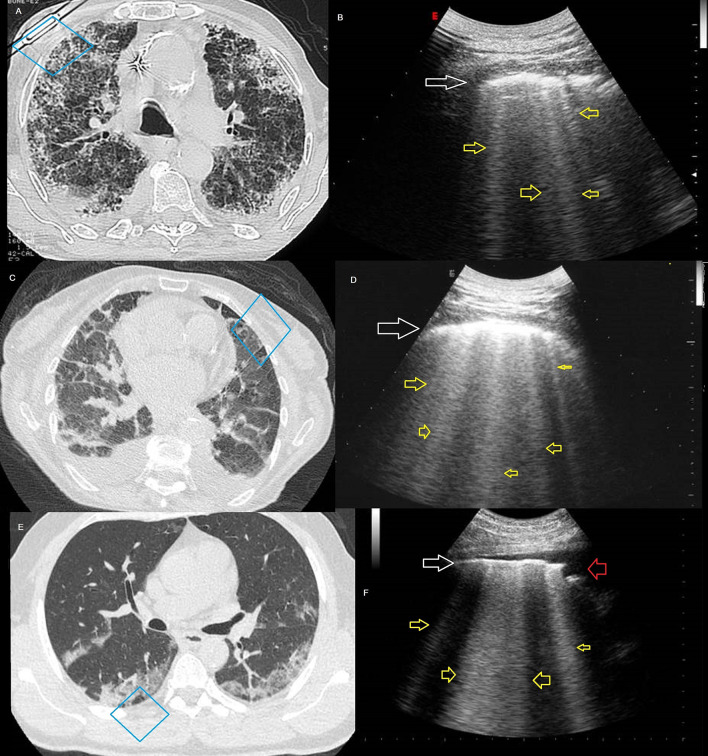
(A) Axial high resolution (HR) CT imaging showing a pattern of non-specific interstitial pneumonia (NSIP). (B) Ultrasound scan (corresponding to the blue box in the A CT scan), with convex probe (6 MHz) and thoracic setting showing irregular thickening of hyperechoic pleural line (white arrow) and B-lines below (yellow arrows). (C) Axial CT imaging showing signs of interlobular interstitial pulmonary edema. (D) Ultrasound scan (corresponding to the blue box in the C CT scan), with convex probe (6 MHz) and thoracic setting showing irregular thickening of hyperechoic pleural line (white arrow) and B-lines below (yellow arrows). (E) Axial CT imaging, in a patient with a fever for 1 week and positive results of RT-PCR assay for the SARS-CoV-2, showing bilateral peripheral ground-glass opacity associated with smooth interlobular and intralobular septal thickening. (F) Ultrasound scan (corresponding to the blue box in the E CT scan), with convex probe (6 MHz) and thoracic setting showing irregular hyperechoic pleural line (white arrow), striated subpleural hypoechogenicity (red arrow), and B-lines below (yellow arrows).
